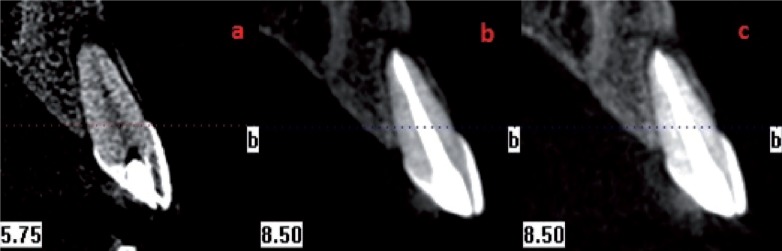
Apical 1/3 and horizontal root fracture. Pretreatment CBCT view (a), post treatment CBCT view (b), control CBCT view (c).
